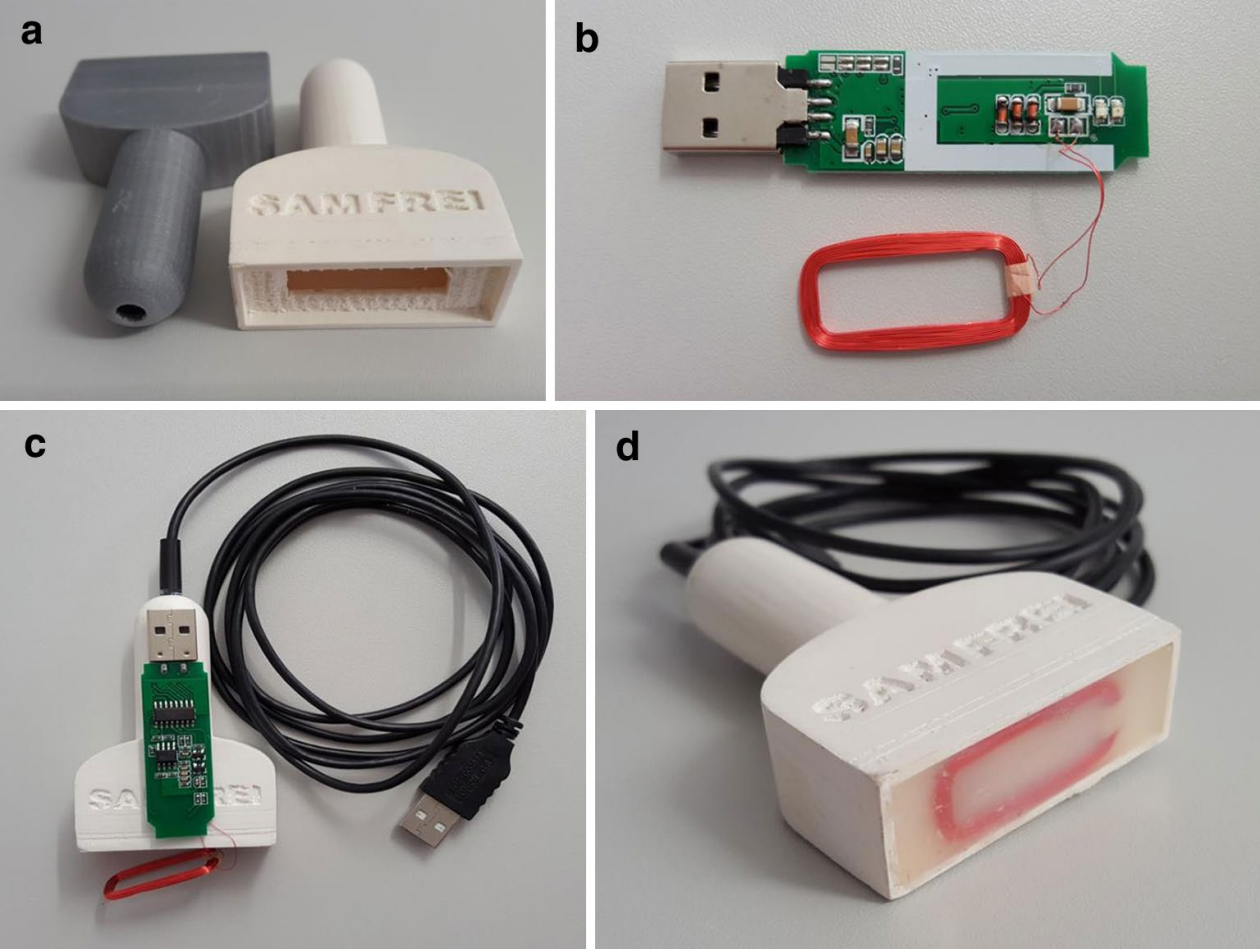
Three-dimensional printed mock ultrasound probe with RFID antenna. a Raw material as printed. b The partly disassembled RFID antenna, which originally has USB-stick format. c How the antenna is built into the housing. d The complete probe with USB wire
